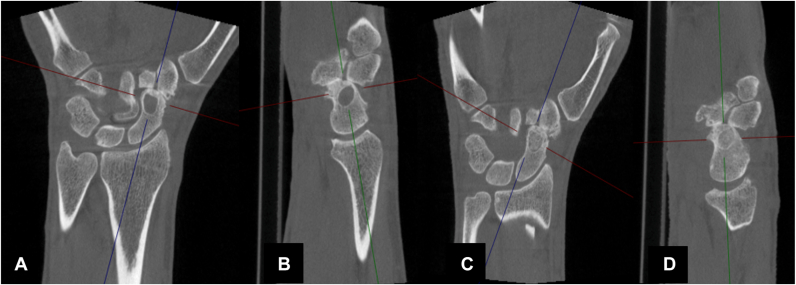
A, B Preoperative CT scan. C, D CT scan 3 months after bone packing. CT, computed tomography.
